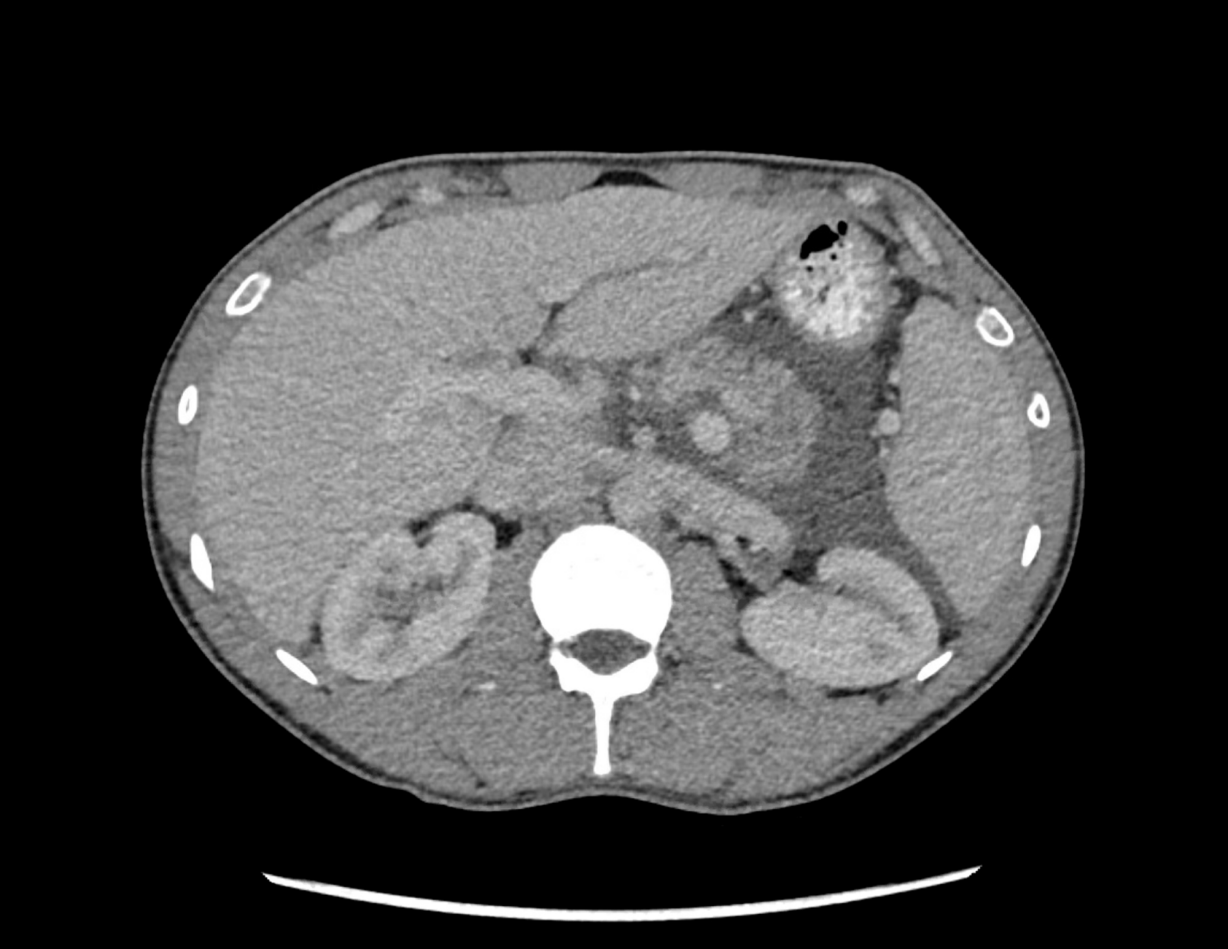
Cross-section of CT scan abdomen showing pancreas with no inflammation CT: computed tomography
